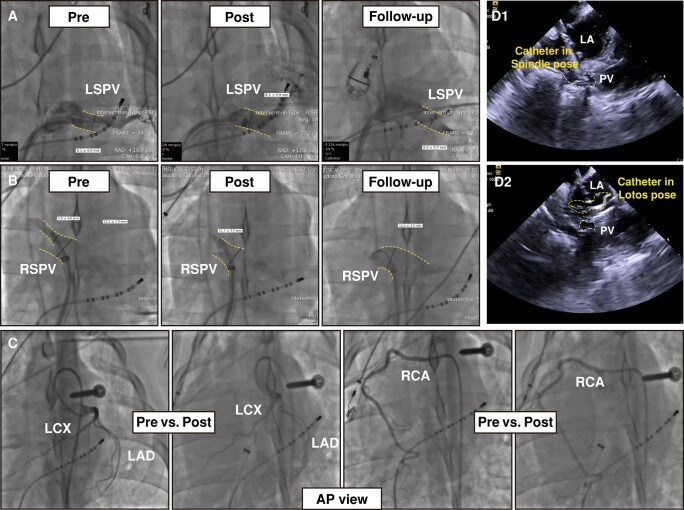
Fluoroscopic views and intracardiac echocardiographic views for safety assessment. (*A* and *B*) Baseline, immediately post ablation, and 30-day follow-up venous angiography of the LSPV (*A*) and RSPV (*B*) showing no venous stenosis. The dotted line denotes pulmonary veins. (*C*) A view of the coronary artery before and 8 min after ablation. (*D*) Intracardiac echocardiographic view of PVs during ablation with spindle pose (D1) and lotos pose (D2), showing no microbubbles. LSPV, left superior pulmonary vein; RSPV, right superior pulmonary vein.
